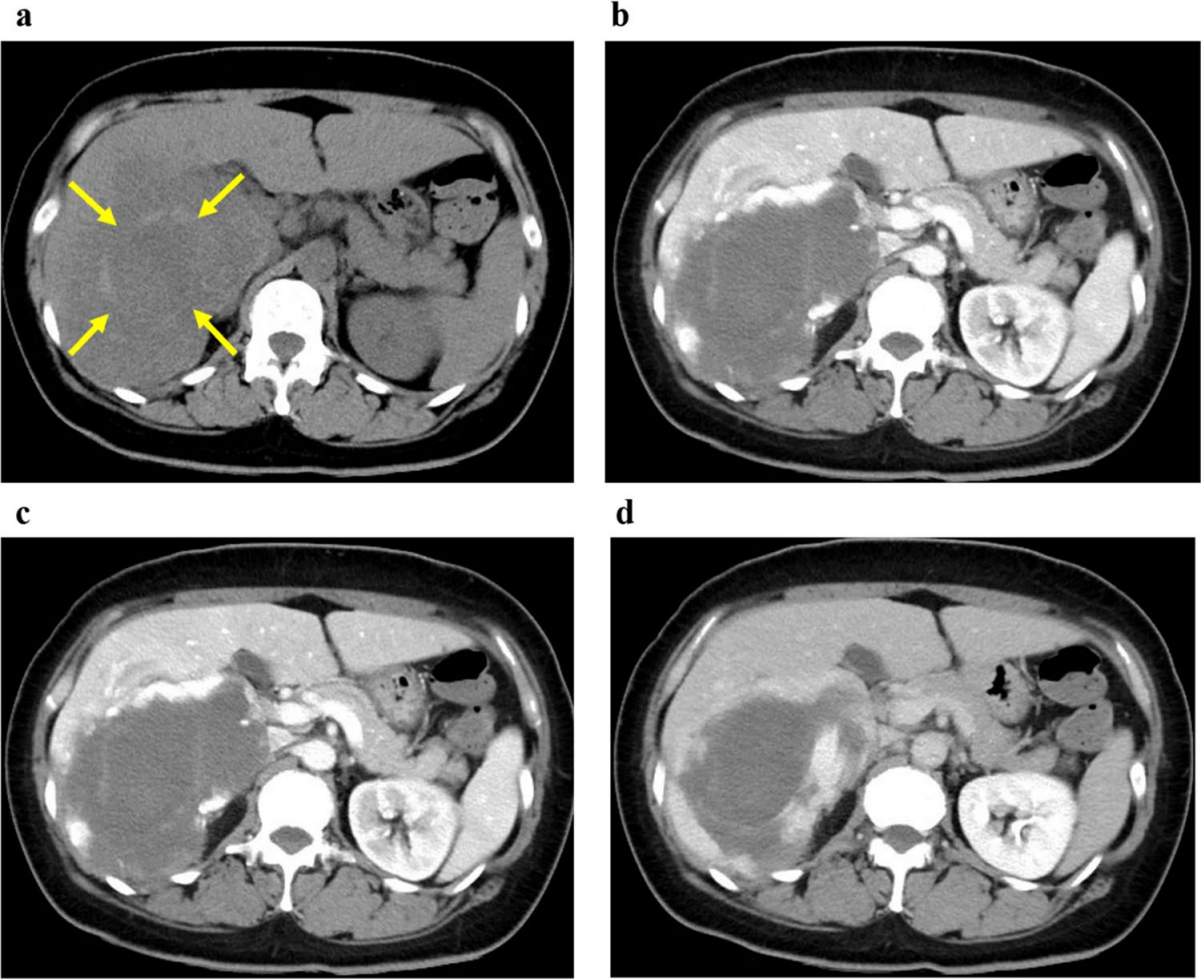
Abdominal contrast-enhanced CT. a Plain. b Artery phase. c Portal phase. d Delay phase d. A high-density area (indicated by yellow arrows) suspicious of hemorrhage and necrosis inside the giant hemangioma, was observed. *CT* computed tomography
